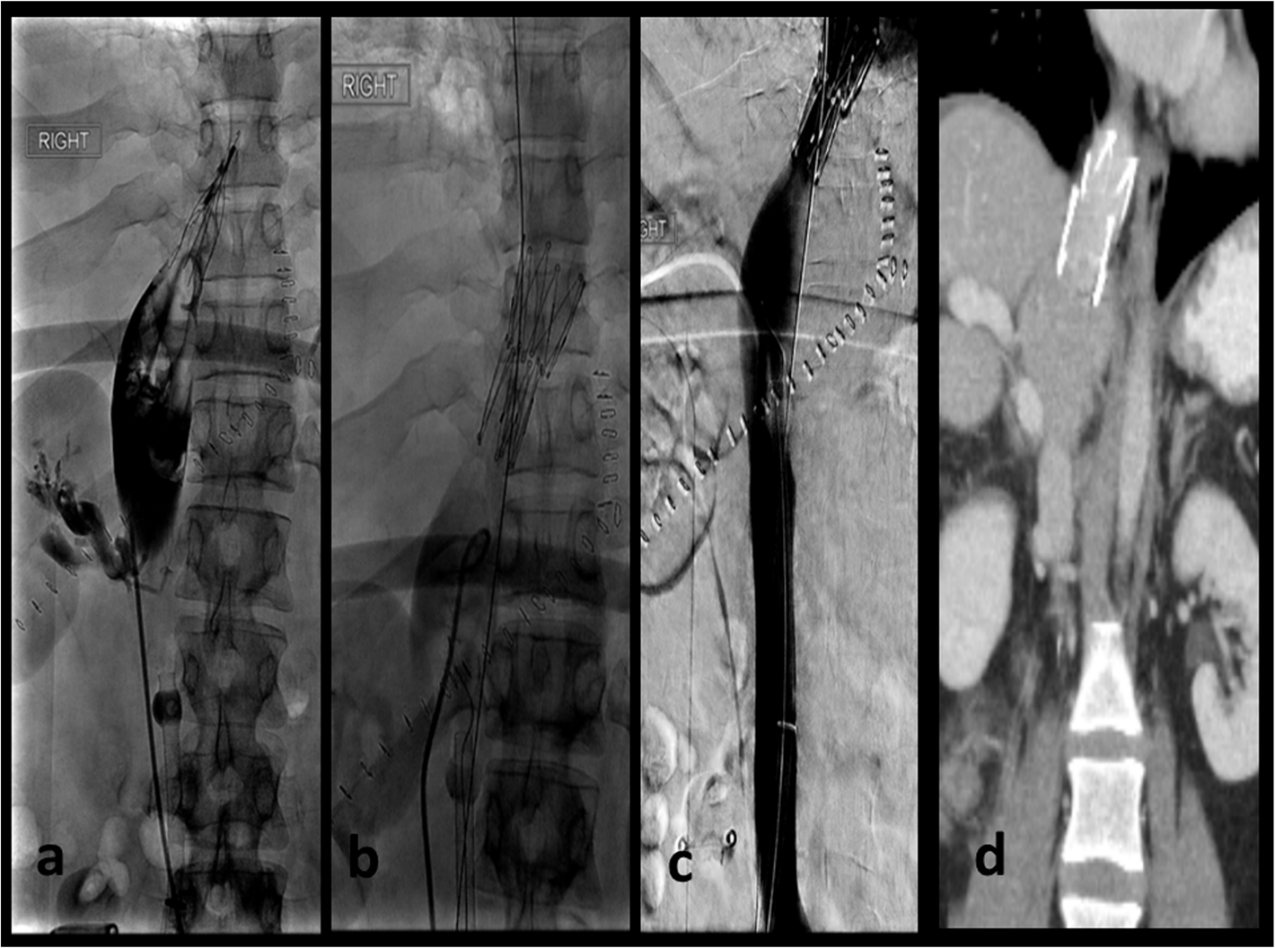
After filter placement, the patient was hypotensive with oliguria and elevated liver enzymes. CT showed extensive thrombus in the IVC. a. and b. Venogram demonstrated large clot burden in the IVC distal to the filter with AngioVac thrombectomy device in place. Z stent was placed at the site of the kinked area. c. and d. Venogram following Z stent placement showed a widely patent IVC. Coronal contrast enhanced CT image of the abdomen demonstrates a patent stent in the intrahepatic IVC
